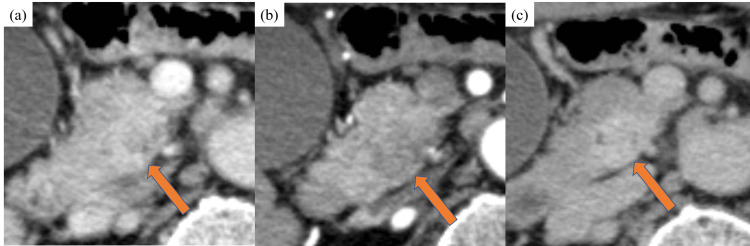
Contrast-enhanced CT images of a 73-year-old man with 26-mm distal bile duct cholangiocarcinoma (arrow) (a) Axial portal phase showing a contrast-enhanced mass with indistinct borders in the pancreatic head. (b) and (c) Axial arterial and delayed phases clearly showing a contrast-enhanced mass between the tumor and pancreatic parenchyma.
